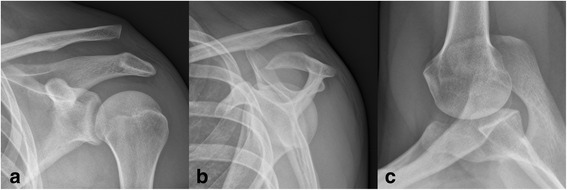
Stress radiography for evaluation of ACJ instability: a Anteroposterior stress radiograph shows complete superior separation in a Rockwood-5 injury. b Alexander stress view shows complete posterior-superior dislocation in a Rockwood-5 injury. c Transaxillary view shows static posterior dislocation in a Rockwood-4 injury
